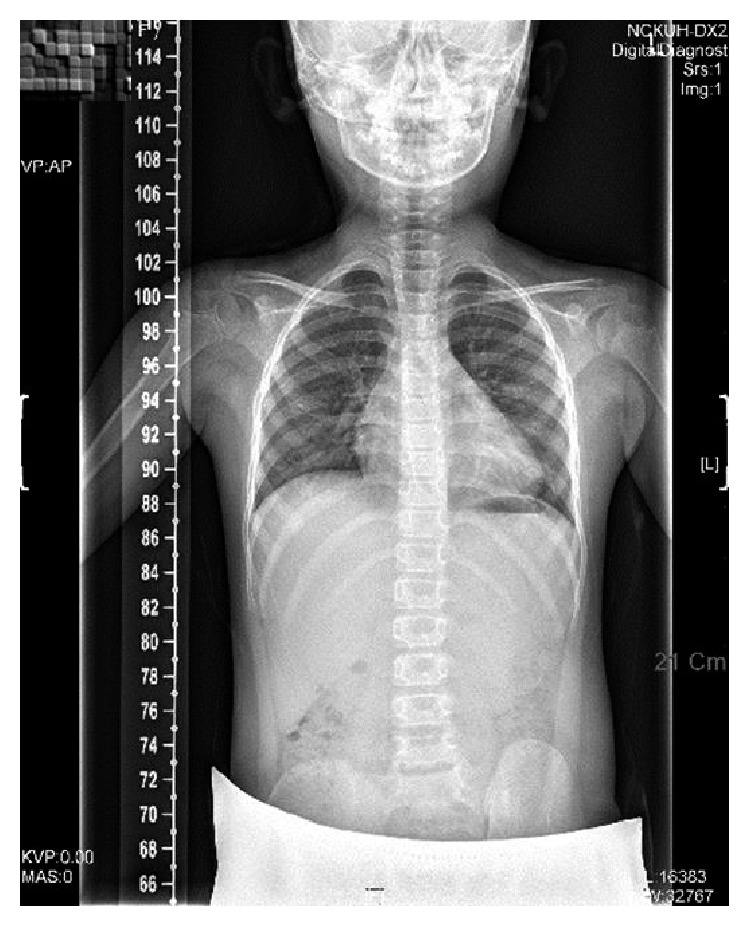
2D X-ray spinal image in the AP view.
